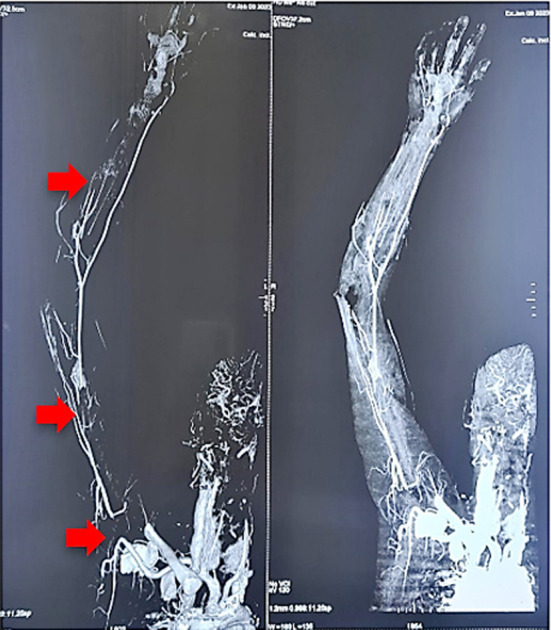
CT angiogram, showing thrombosed axillary, brachial and ulnar arteries.
